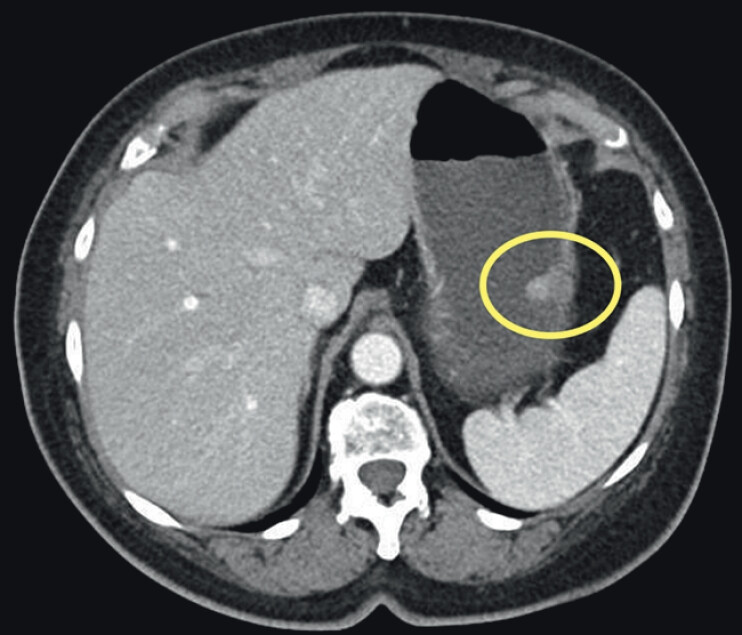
Computed tomography of the abdomen/pelvis revealing a 2.5-cm gastrointestinal stromal tumor (GIST) localized to layers 3 and 4.
